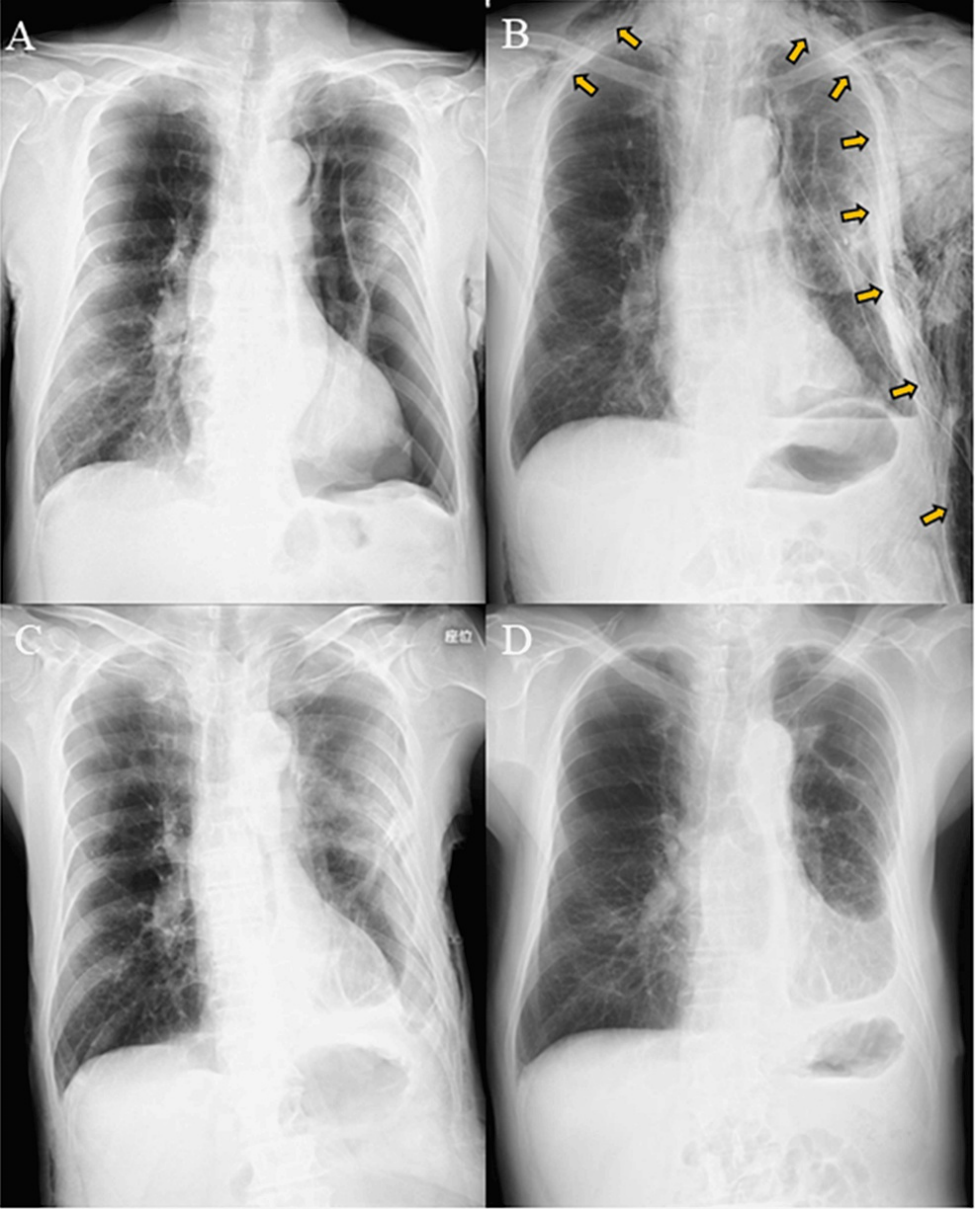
Chest radiography on (A) admission, (B) postoperative day 1, (C) day 9, and (D) day 30
